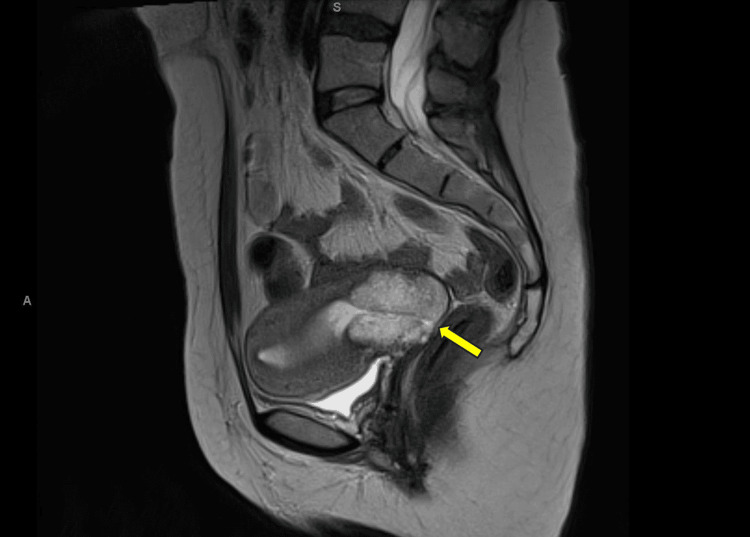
Sagittal T2 MRI. The yellow arrow is pointing to the cervical lesion.
